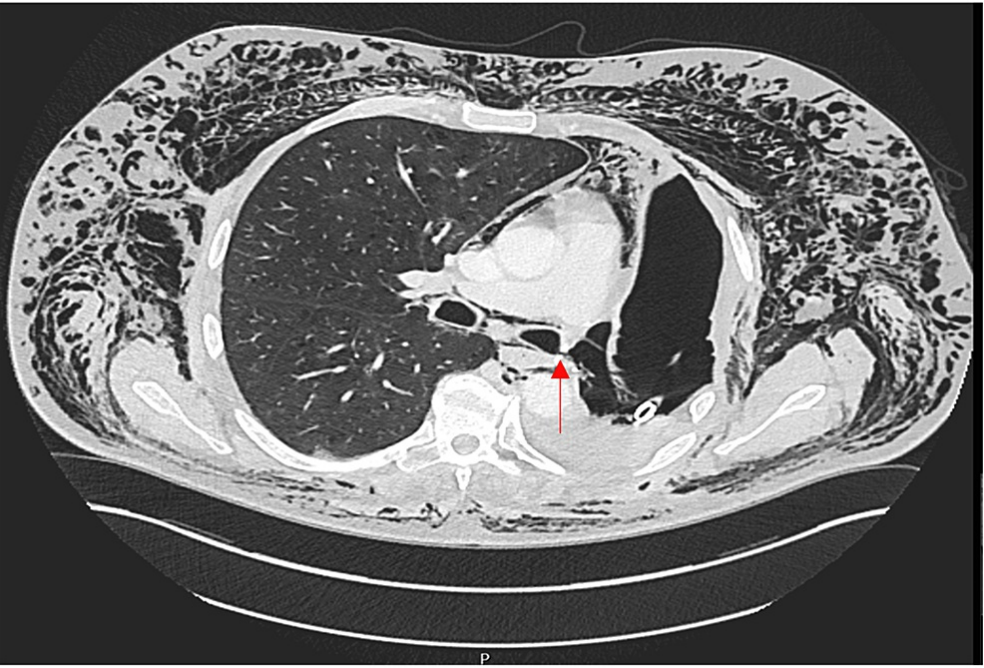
CT scan demonstrating the presence of a bronchopleural fistula in the posterior edge of the left bronchial stump following pneumonectomy (arrow) CT: computed tomography
